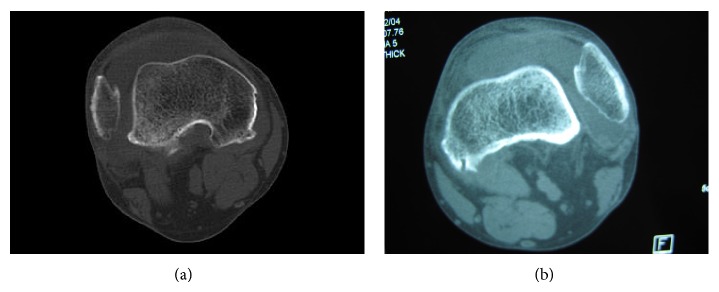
CT images of right (a) and left (b) knees.
